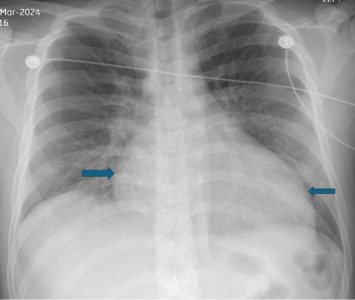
Chest X-ray image showing cardiomegaly.
